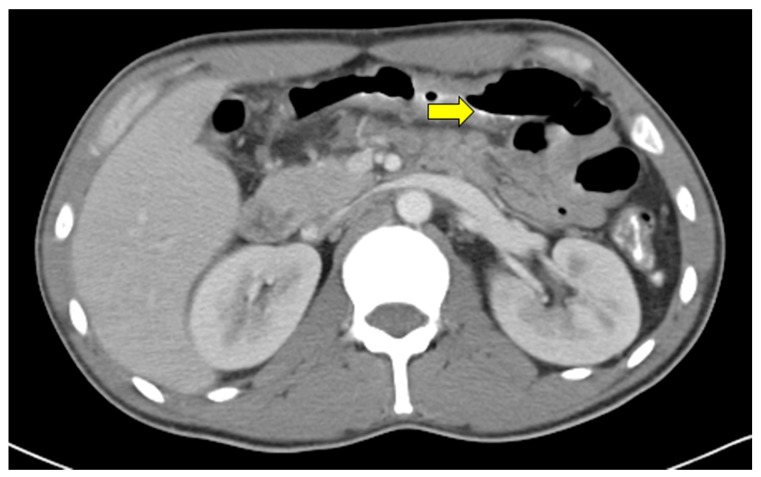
Abdominal computed tomography scan. The yellow arrow points to the wall thickening of the transverse colon.
